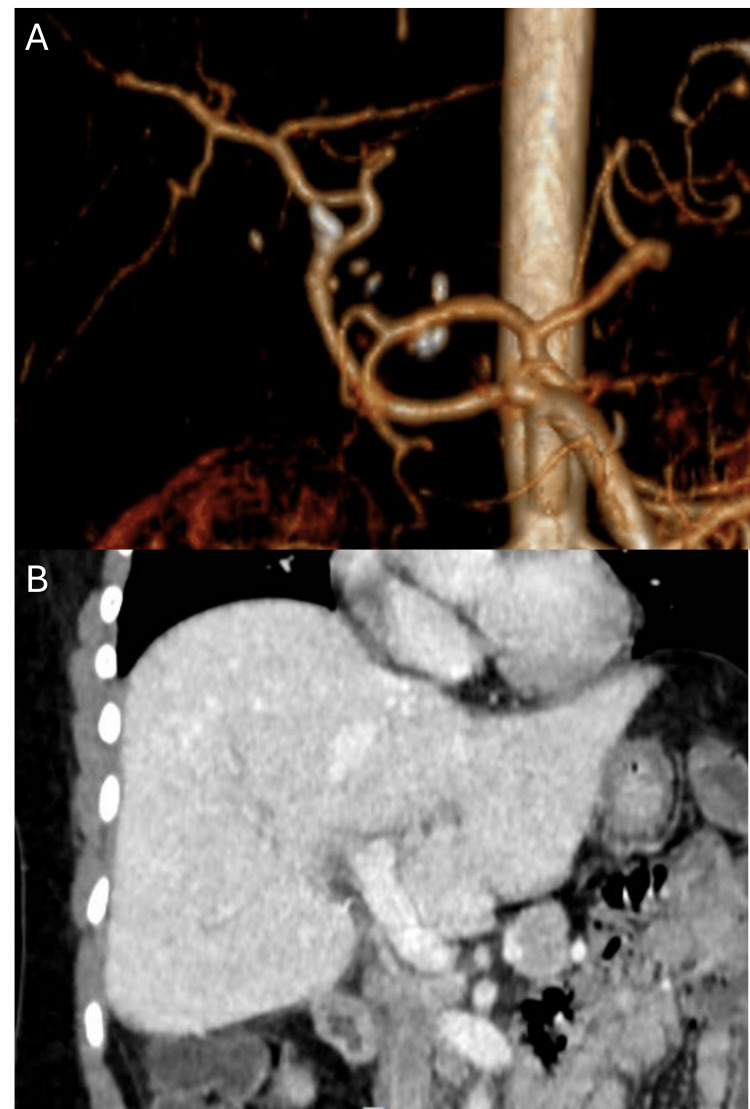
Postoperative abdominal computed tomography with intravenous contrast one year after transplantation. (A) Digital reconstruction of the arterial phase showing a variant right hepatic artery and a patent anastomosis of the graft’s proper hepatic artery. (B) Coronal reconstruction of the portal phase showing a normal liver graft with a patent portal vein.
